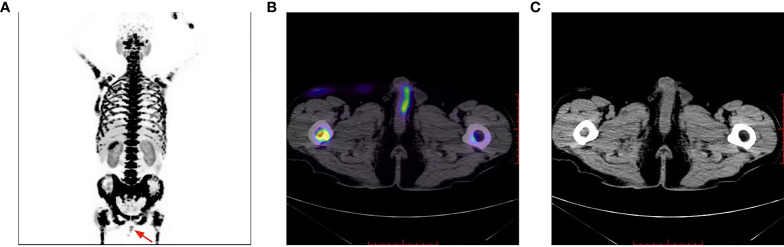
Penile lesion was enlarged with a higher SUV_max_ of 7.4 (B); meanwhile, systemic bone metastasis was certifified (A). No morphological abnormalities of the penis detected on CT imaging (C).
